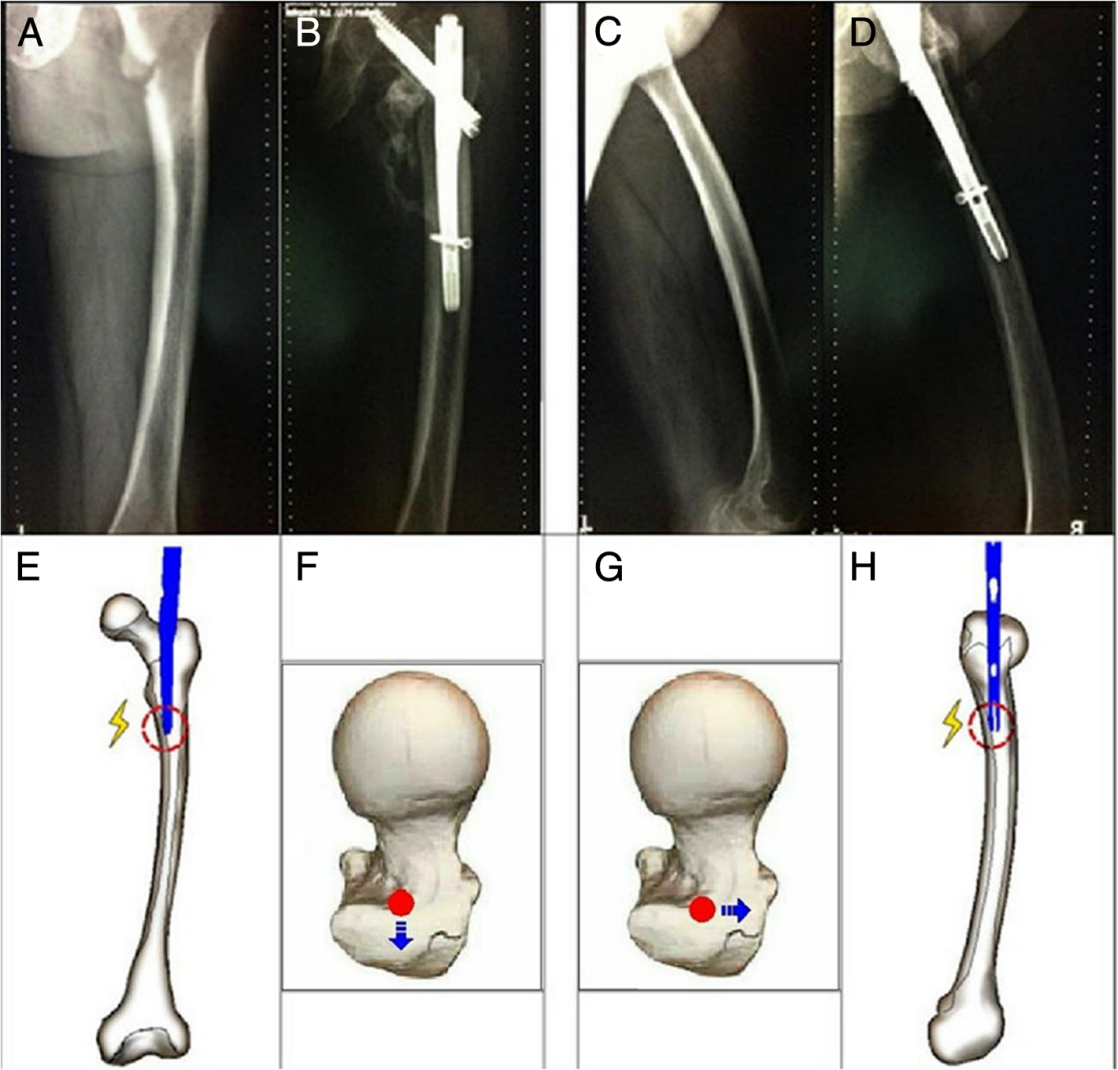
Anterior, posterior, and lateral x-ray images of full length of the injured femur during & after two months surgery with femoral anatomical specificity of antecurvation. (A) Full-length anteroposterior X-ray image of the injured femur during the operation. (B) Full-length anteroposterior X-ray image of the injured femur two months after surgery. (C) Full-length lateral X-ray image of the injured femur during the operation. (D) Full-length lateral X-ray image of the injured femur two months after surgery. (E) If the patient has the anatomical specificity of lateral curvation of the femoral shaft, resistance will be encountered while implanting the nail at the routine entry point. (F) Accordingly, the entry point should shift to the lateral. (H) If the patient has the anatomical specificity of antecurvation of the femoral shaft, resistance will be encountered while implanting the nail at the routine entry point. (G) Accordingly, the entry point should shift forward.
